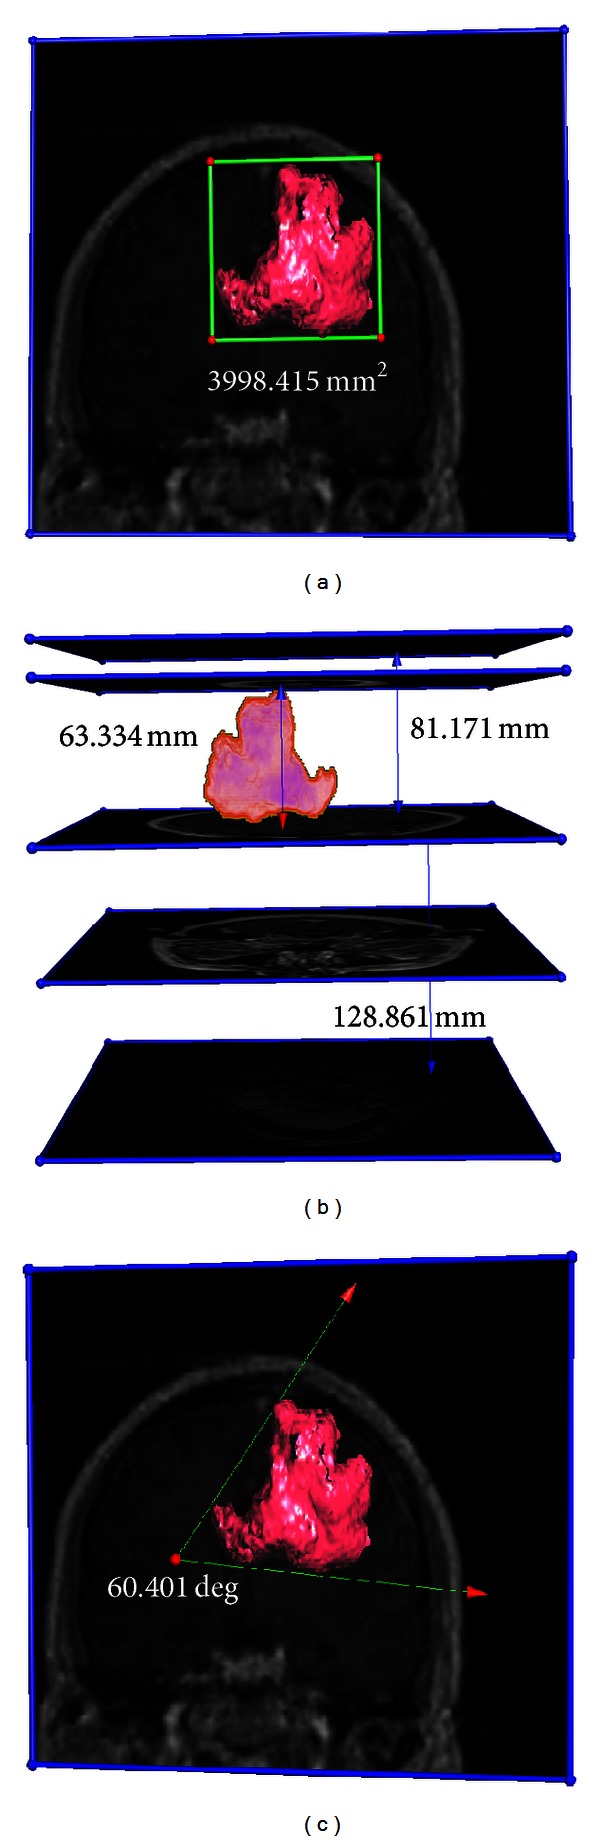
Interactive 3D measurement of the tumor in the brain MR image. First, the tumor is segmented from the MR image using a region growing method automatically. Then, the tumor model and the original MR image are put in the same rendering environment for interactive 3D measurement. (a) Area measurement (surface model of the tumor is used and rendered by surface rendering). (b) Distance measurement (volume model of the tumor is used and rendered by ray casting). (c) Angle measurement (surface model of the tumor is used and rendered by surface rendering).
